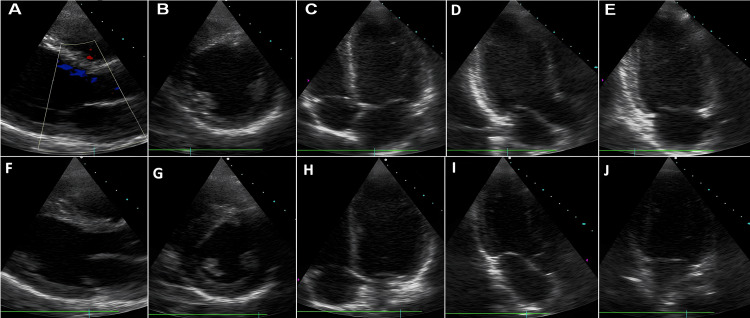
Two-dimensional echocardiographic images in the acute phase (upper panel) and after recovery (lower panel). Generalized severe hypokinesia, dilatation of the left ventricle, and low LVEF in the acute phase were the major findings (A-E). Three days after the episode, the heart was fully recovered (F-J). A and F show parasternal axis, B and G show short axis, C and H show apical four-chamber view, D and I show apical three-chamber view, and E and J show apical two-chamber. LVEF: left ventricular ejection fraction
